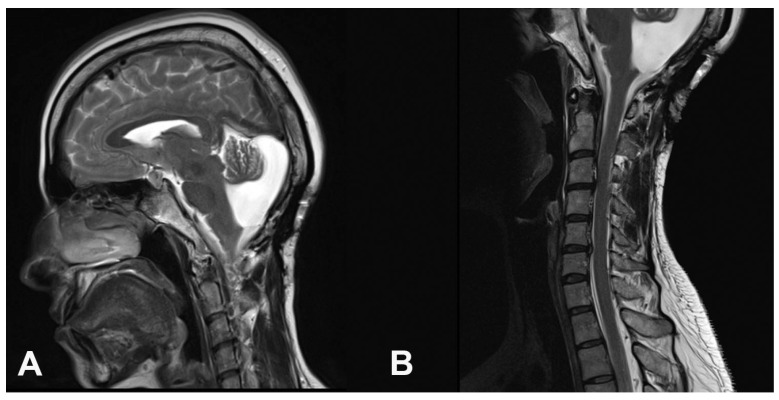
Follow-up MRI six months after the surgery showed a decrease in the size of the posterior fossa cyst (A) and complete resolution of the syrinx (B).
